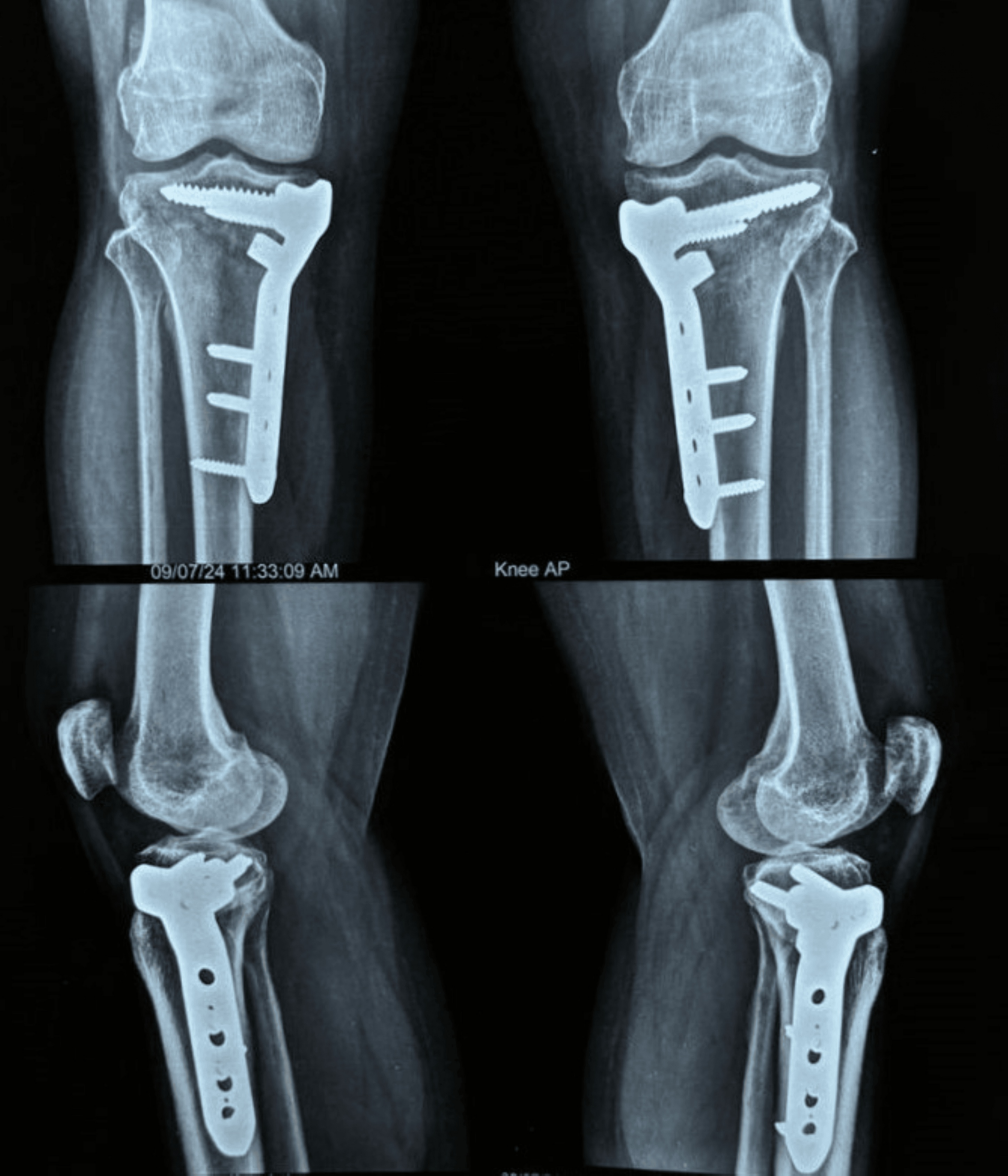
One-year follow-up X-ray showing healing of the osteotomy site with intact lateral hinge.
